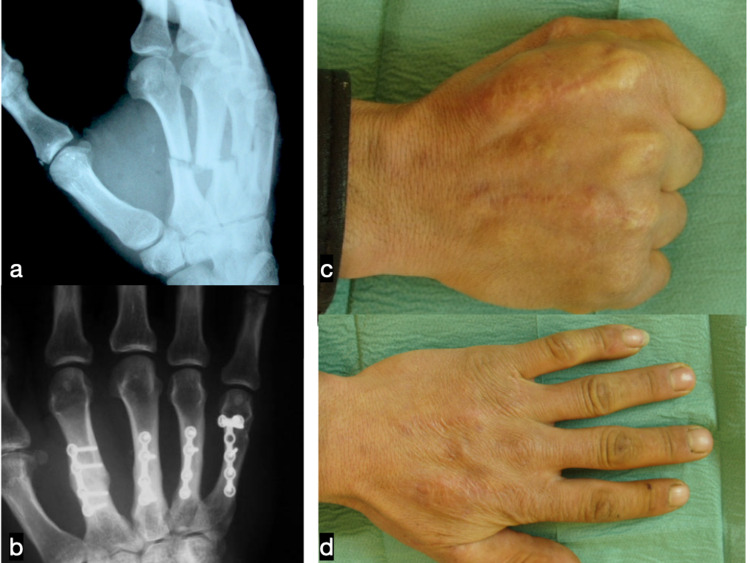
Preoperative radiograph of a patient who sustained closed fractures of the second through the fifth metacarpal (a). He underwent plate fixation of all fractures (b). Range of motion is presented six years after treatment (c,d)
